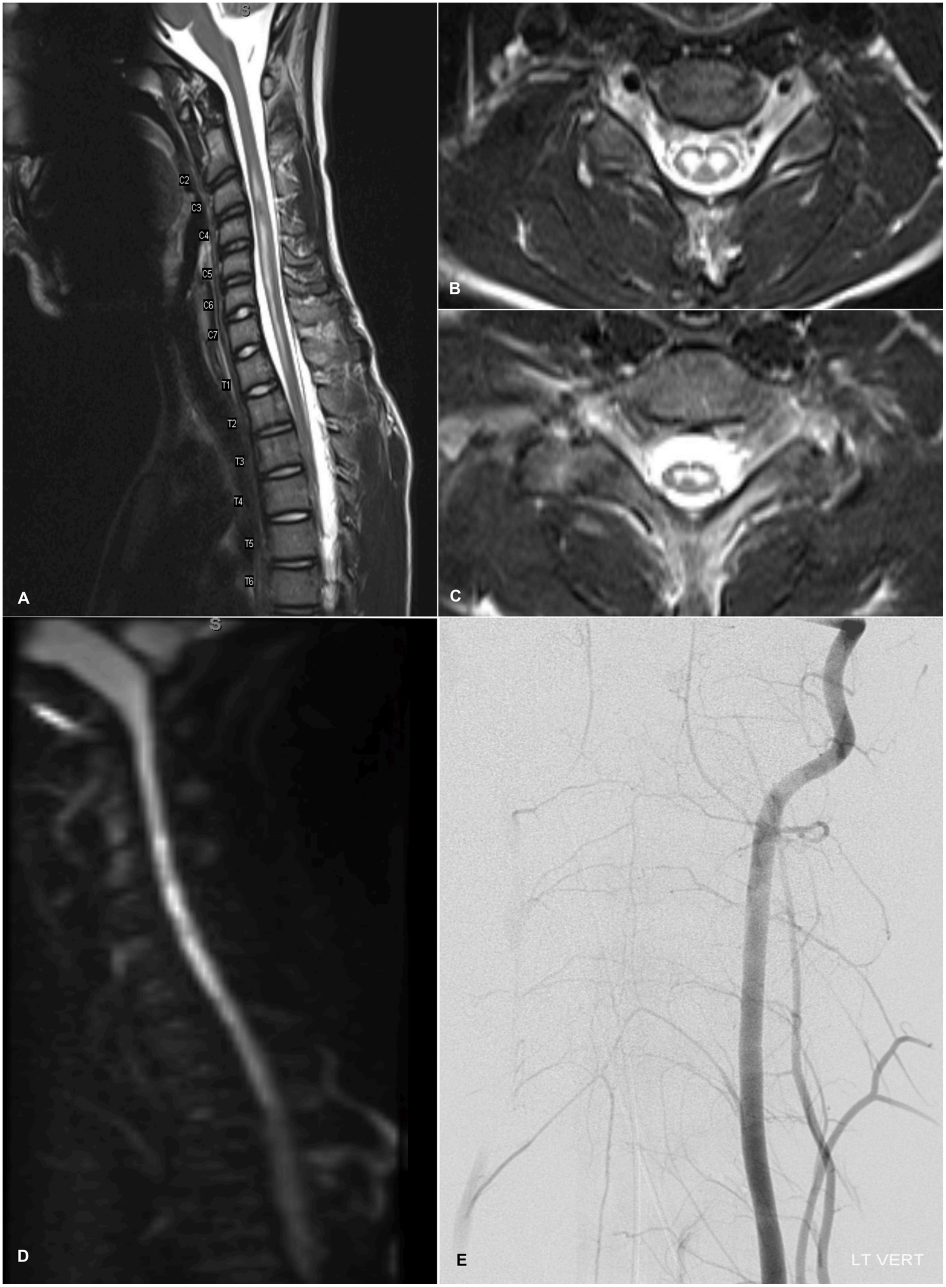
(A) T2-weighted sagittal admission MRI image of the cervical spine showing longitudinally extensive, non-enhancing, mildly expansile hyperintensity within the central gray matter of the spinal cord spanning levels C2-T2 with normal appearance of the vertebral flow voids. Mild intervertebral disc intensity changes are observable at C2-C6. (B,C) Axial T2-weighted MRI images at levels C6 and C8 respectively demonstrating localization of T2-hyperintensity to the anterior horns with an “owl's eye” appearance. (D) Diffusion-weighted imaging demonstrating diffusion restriction within the cervical cord lining up with the T2-hyperintense lesion. (E) Diagnostic angiogram showing the cervical view of the left vertebral artery catheterization. Brisk and robust collateralization to the vascular supply of the spinal cord, including the anterior spinal artery, and spinal canal is seen. There is no evidence of infarction or dissection of the spinal vasculature.
